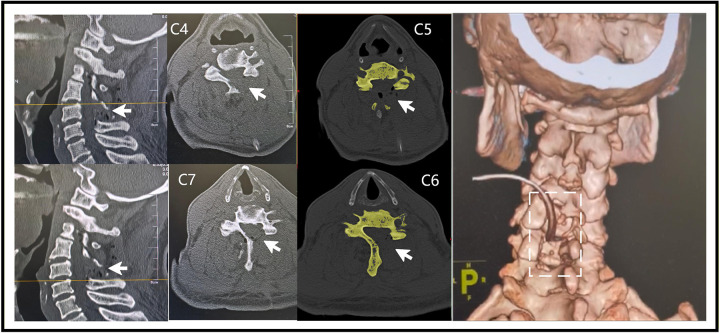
Postoperative CT of the patient demonstrating: Complete laminectomy at C5; Undercutting decompression via hemilaminectomy at C4, C6, and C7.
